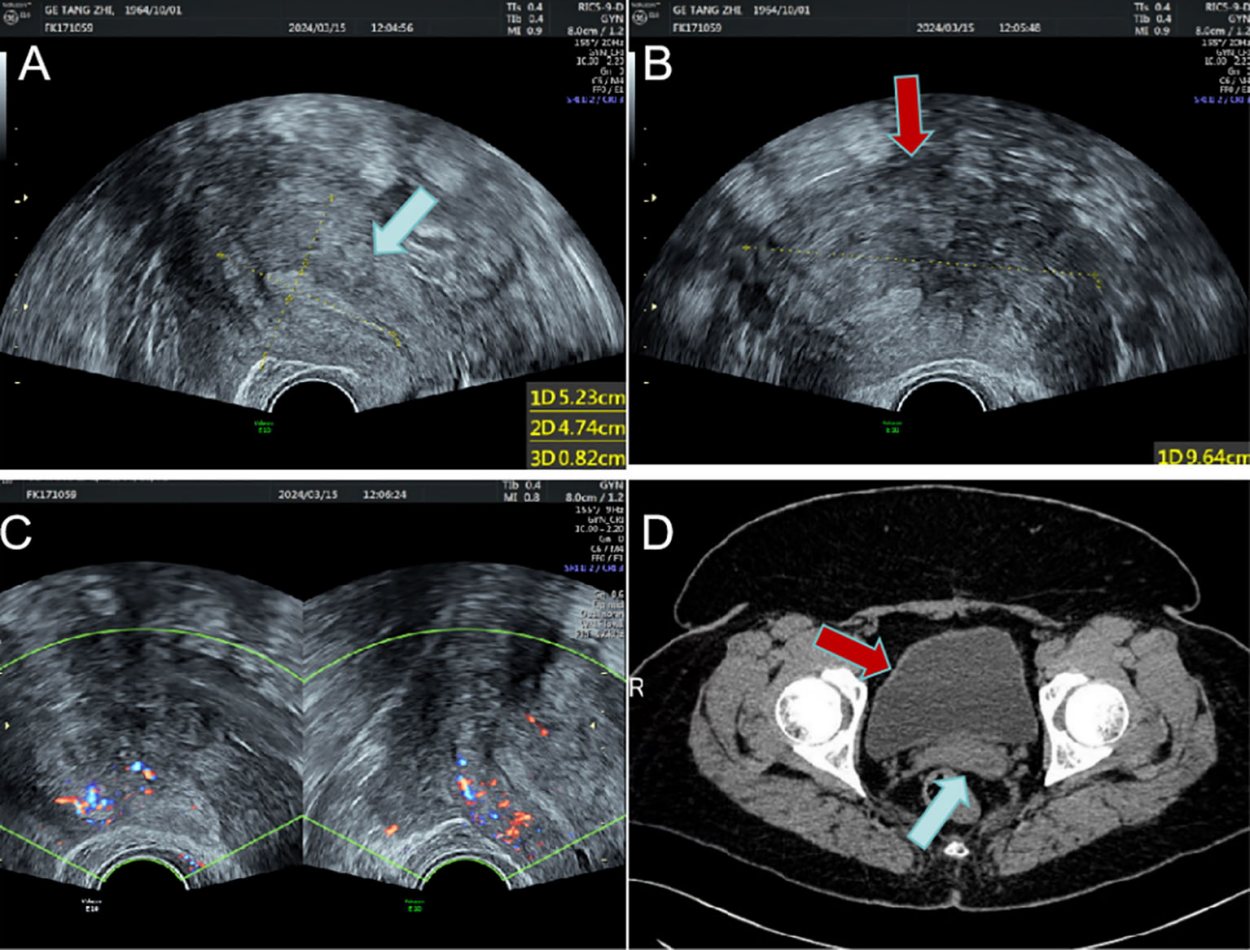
The uterus and the huge mass are shown on imaging. (A) Enlarged uterus on transvaginal sonography(blue arrow); (B) Large hypoechoic mass around the uterus(red arrow); (C) Blood flow signals around the uterus; (D) The uterus (blue arrow) and surrounding giant mass (red arrow) on enhanced CT.
